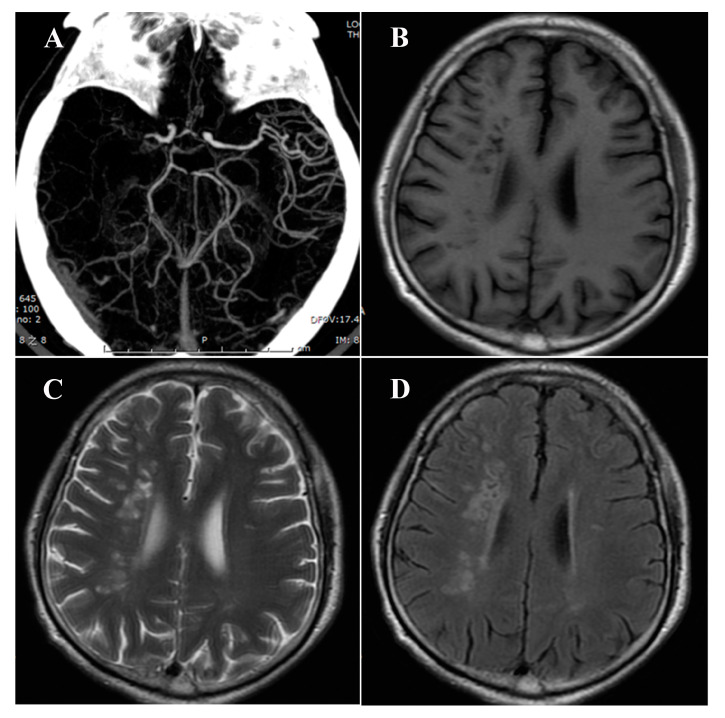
One representative case of interhemispheric differences of CSVD. Note: (A) Computed tomographic angiography shows occlusion of the right MCA in the M1 segment. (B–D) T1-weighted, T2-weighted, and T2-weighted fluid-attenuated inversion recovery images show increased lacuna number in the hemisphere ipsilateral to the MCAO compared with the contralateral hemisphere.
